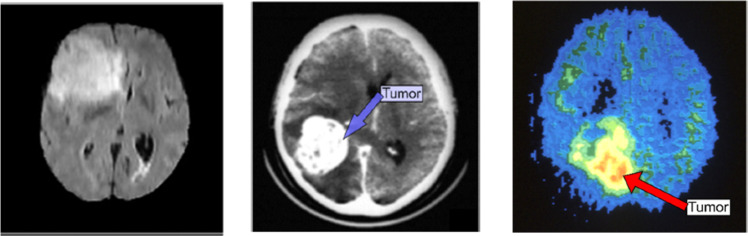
Brain tumor identification using (a) MRI, (b) CT, (c) PET [14].
